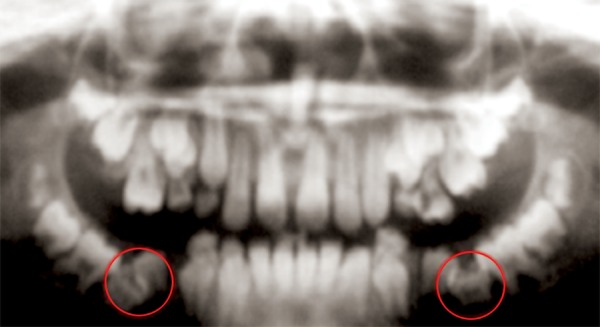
Panoramic radiograph showing bilateral submerged primary second molars
